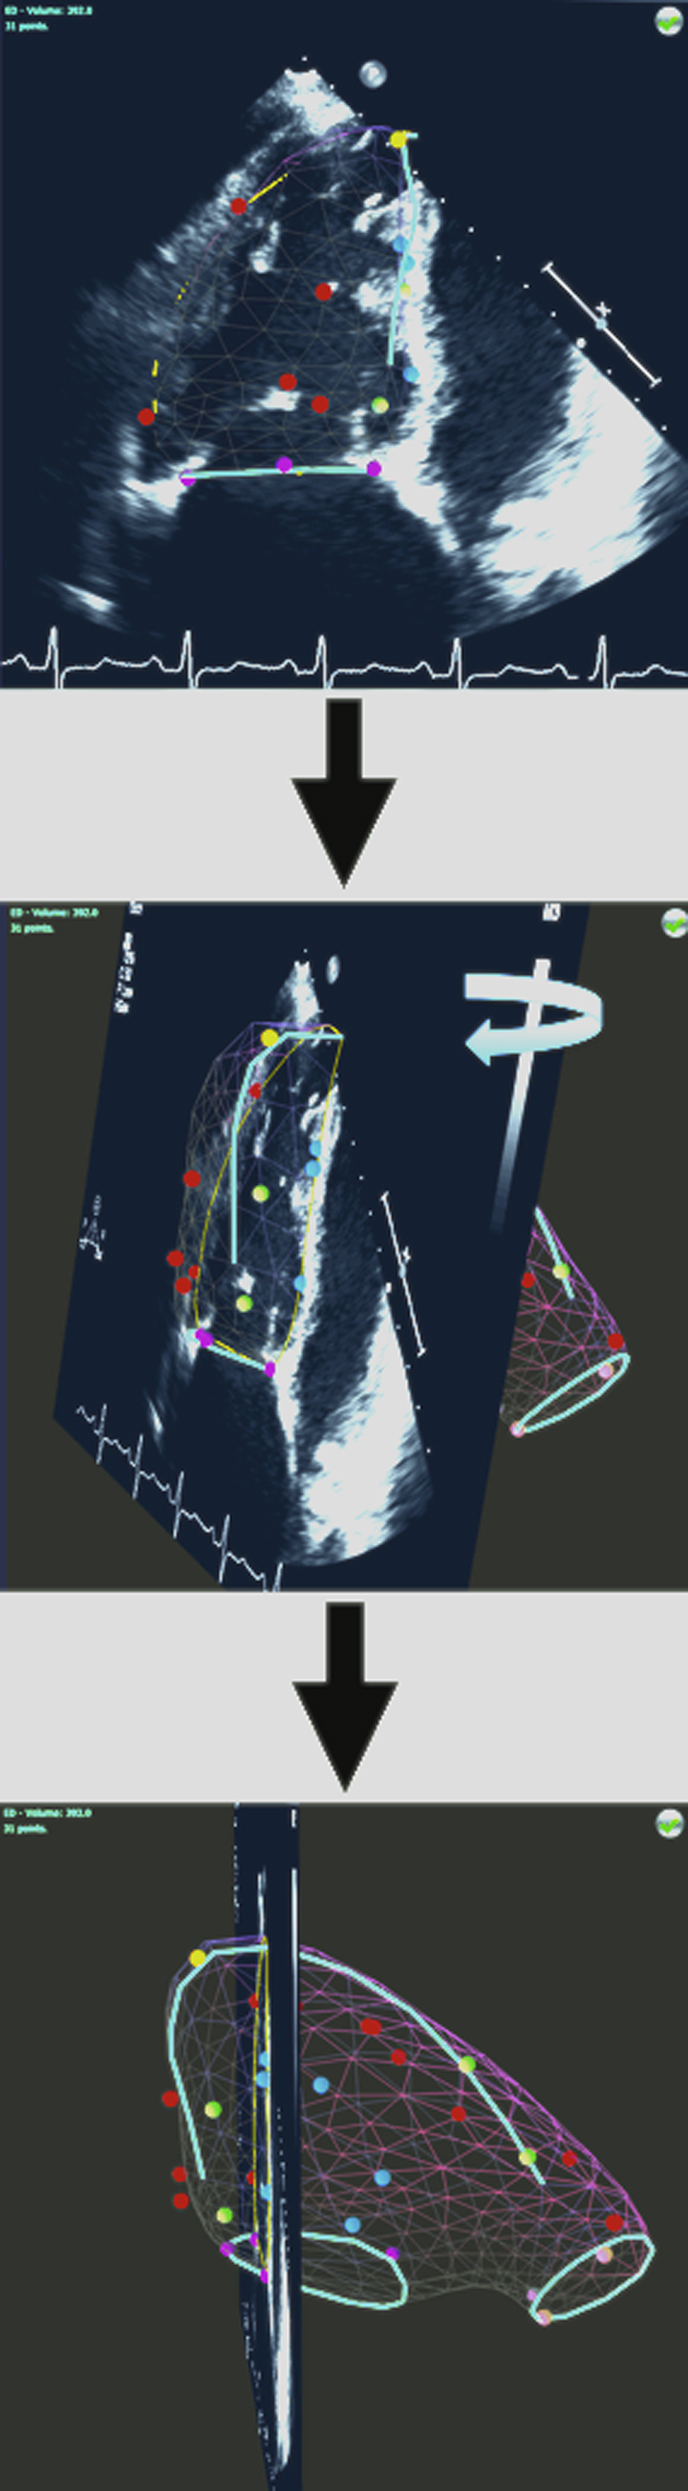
Demonstration of the interaction between the reconstructed 2D knowledge-based reconstruction polygon with a four-chamber view 2D echocardiographic scan plane. The reconstructed polygon can be rotated in any direction (here through 90° from top to bottom, indicated by the curved arrow). Any original 2D echocardiographic acquisition can be displayed (here, the four-chamber view) and viewed in relation to the polygon by clicking on one of the dots. In this way, the reconstructed polygon can be inspected to ensure accurate alignment with the original 2D echocardiographic data. From this view, it is also readily appreciable how much of the right ventricle, predominantly the outflow portion, is neglected in a standard four-chamber view used to derive fractional area change.
